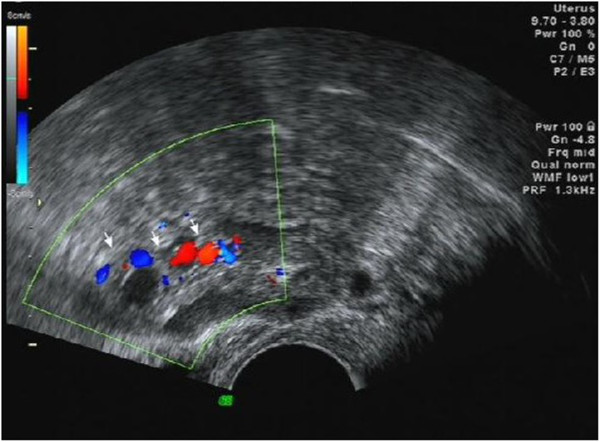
An ultrasound scan (on 16 December 2013) showed a 24mm×14mm left adnexal mass.
